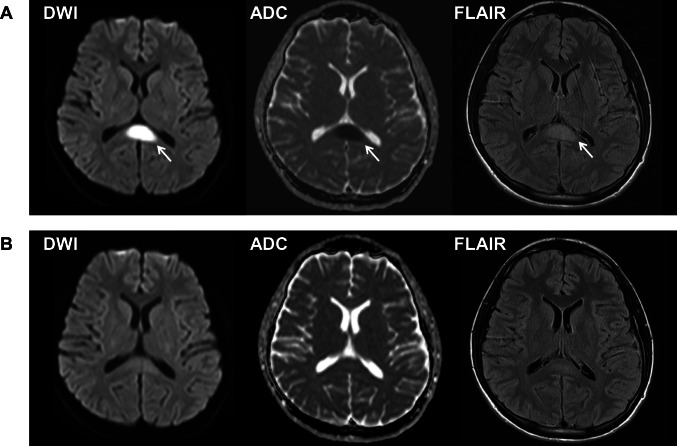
Magnetic resonance images of the brain of Patient 1 on Day 18 (A) and on Day 25 (B) after COVID-19 vaccination. A Diffusion-weighted image (DWI) (left) shows restricted diffusion in the splenium with low apparent diffusion coefficient (ADC) values (middle), and fluid-attenuated inversion recovery image (FLAIR) (right) shows a high signal intensity lesion at the midline of the splenium of the corpus callosum (arrows). B The lesion disappeared after intravenous high-dose methylprednisolone therapy
